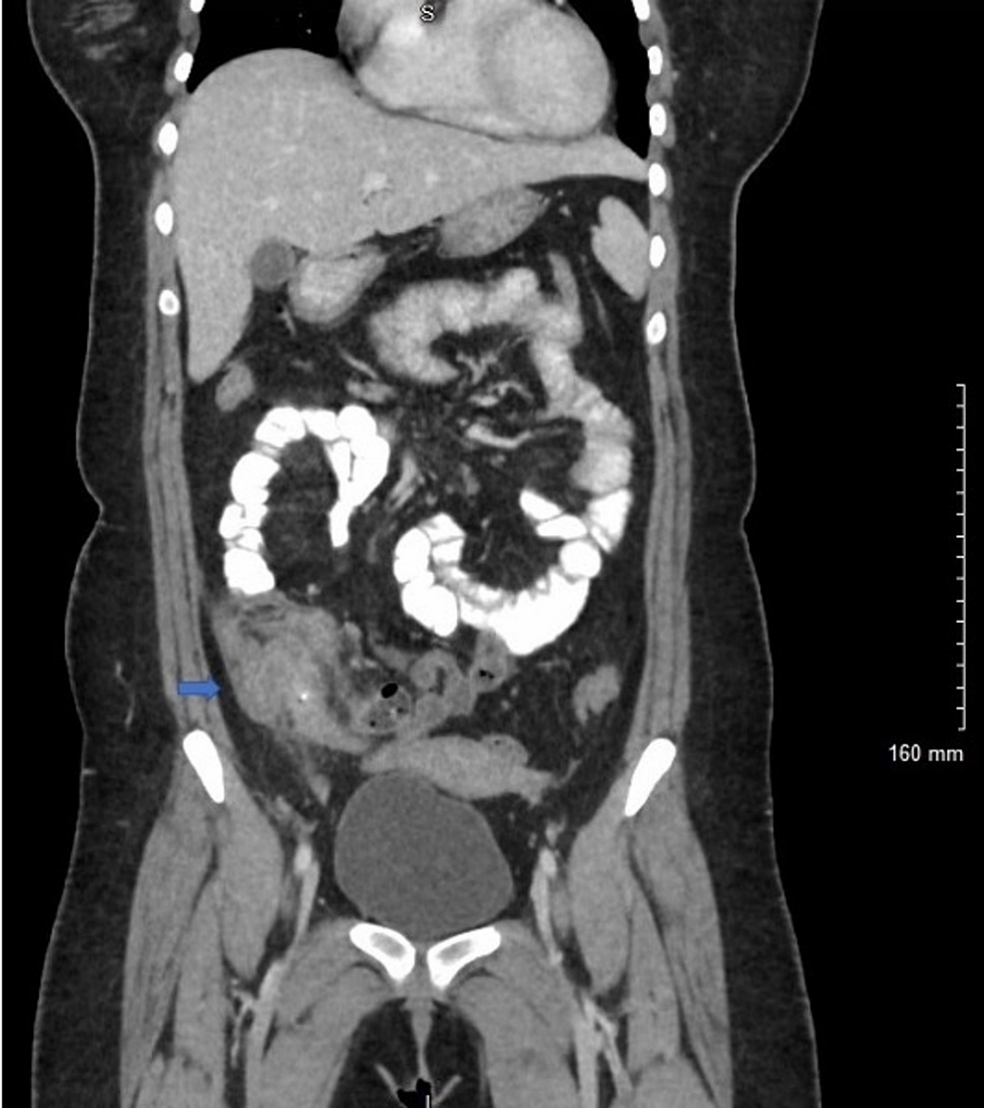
CTAP with contrast: coronal view demonstrating inflammatory thickening of cecum CTAP, Computed tomography arterial portography.
